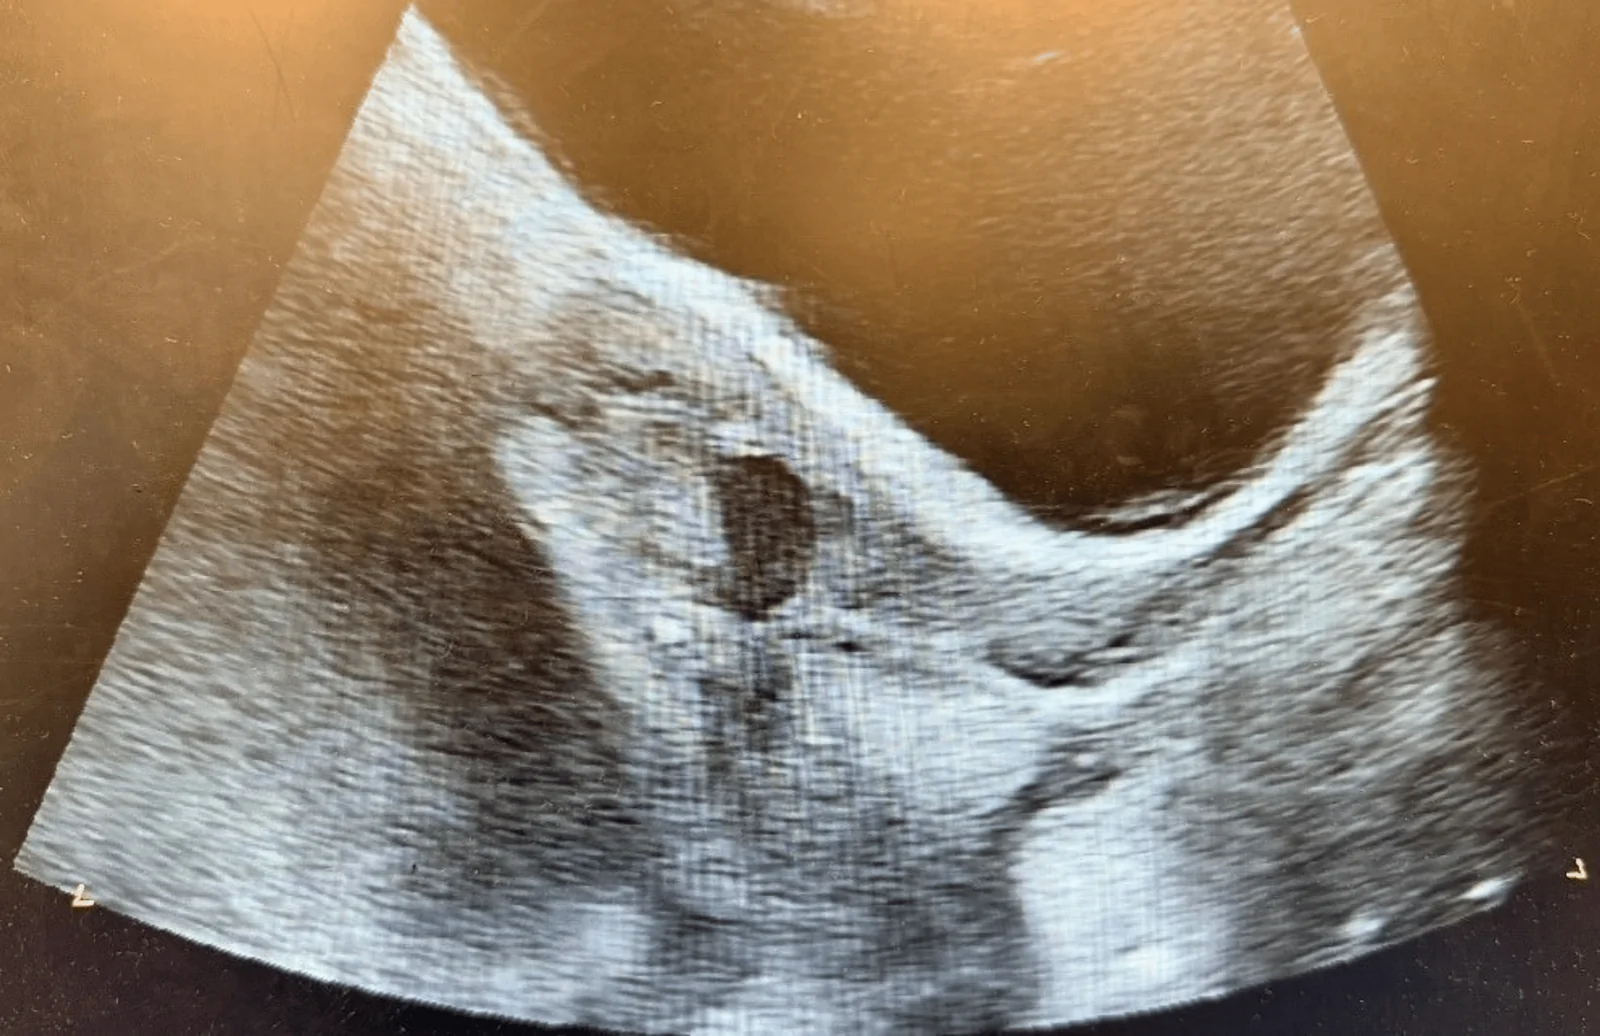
Pelvic ultrasound showing bicornuate uterus with IUCDs in situ No ovarian or pelvic pathology is observed IUCDs: intrauterine contraceptive devices
